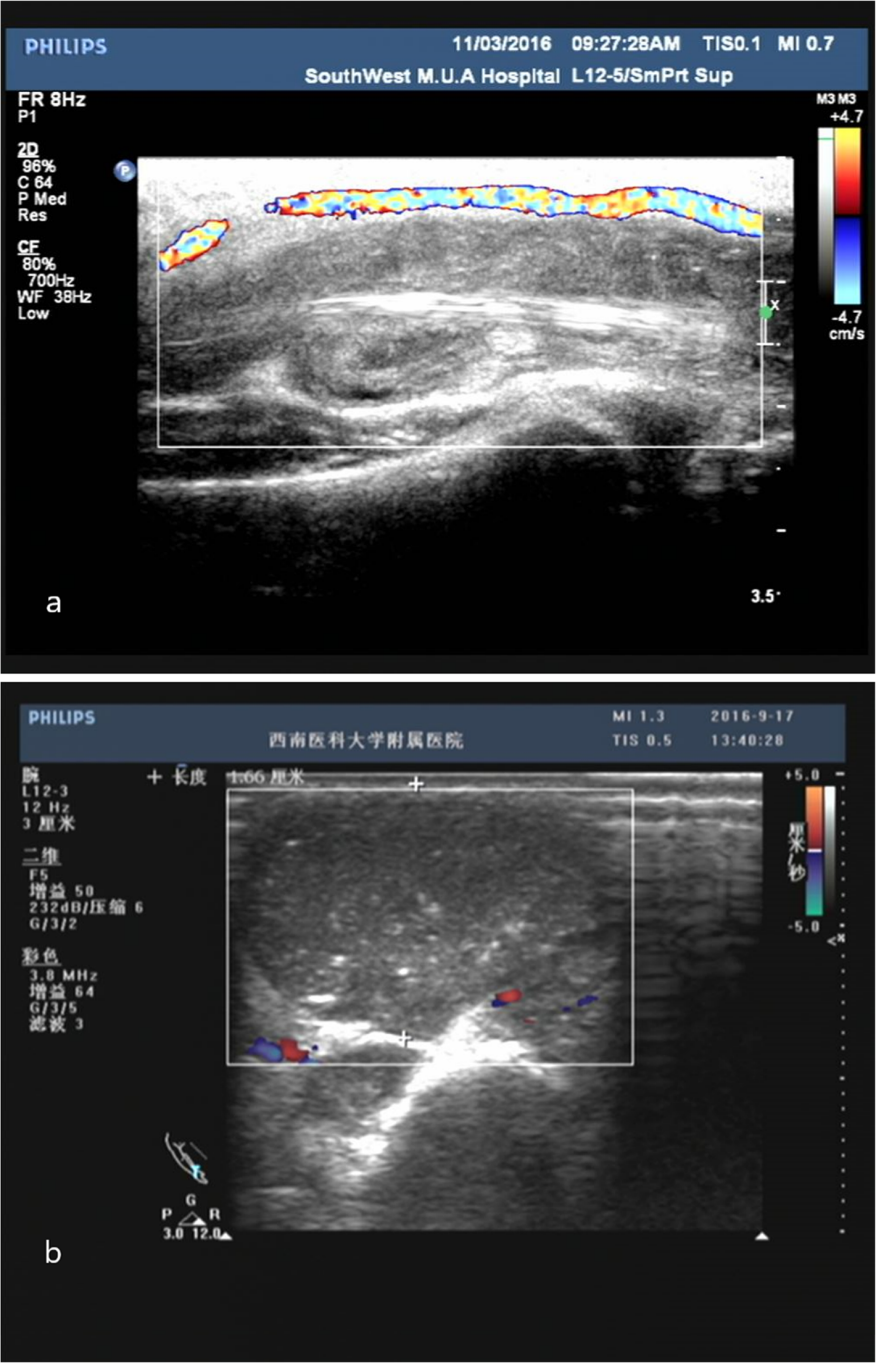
a, b Ultrasonography showing a cystic mass of the wrist with clear boundaries and an irregular shape, in which hyperechoic echoes can be seen
